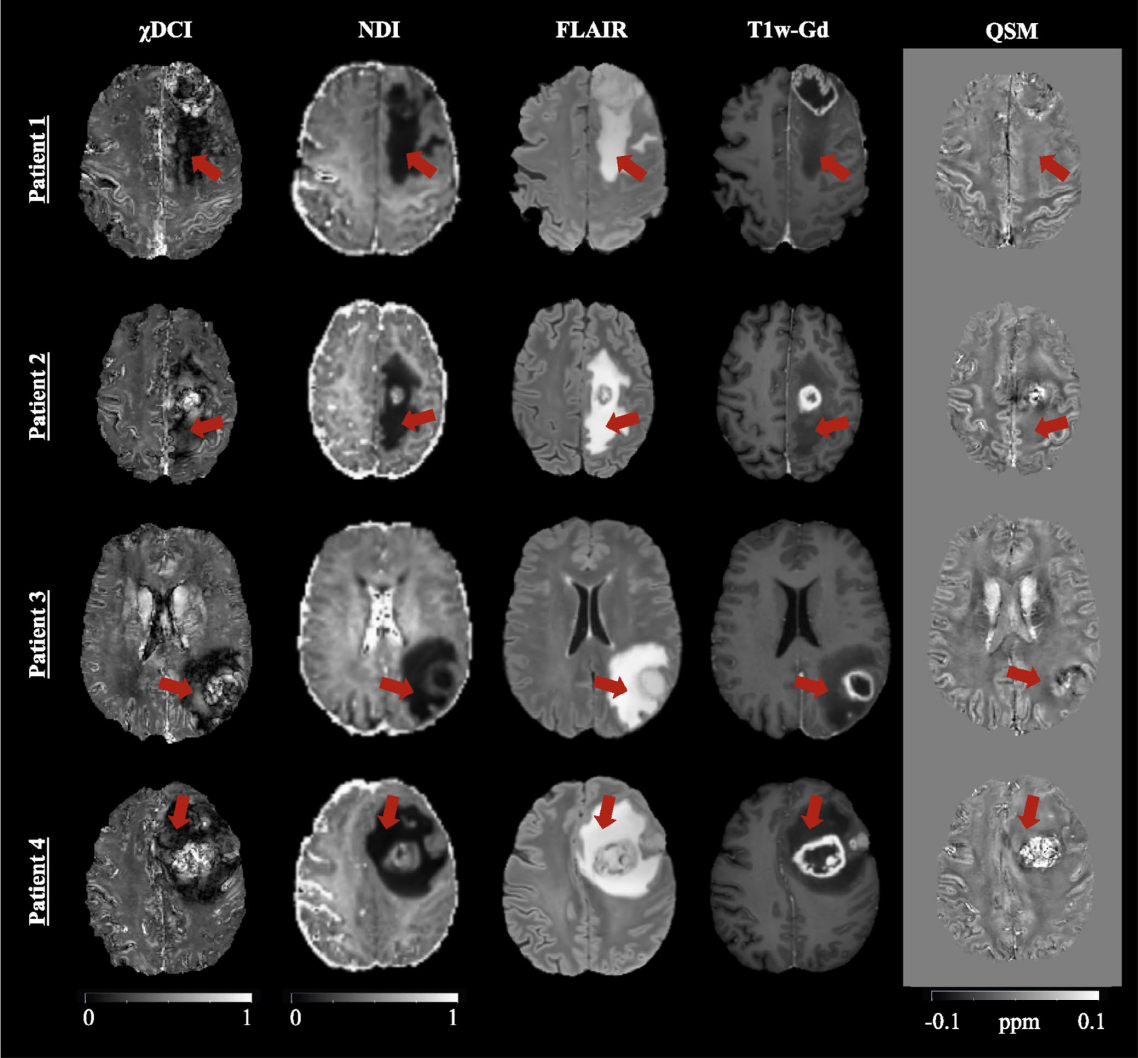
Examples of images of four patients with glioblastoma. From left to right: χDCI, NDI, FLAIR, T1w‐Gd and QSM. Heterogeneity within the different tumor regions (i.e., contrast enhancement, necrosis and edema) can be appreciated on χDCI but not on NDI. For visualization purposes, all images are warped to the χDCI native space. Red arrows point at a location in which the edema is heterogeneous on χDCI.
